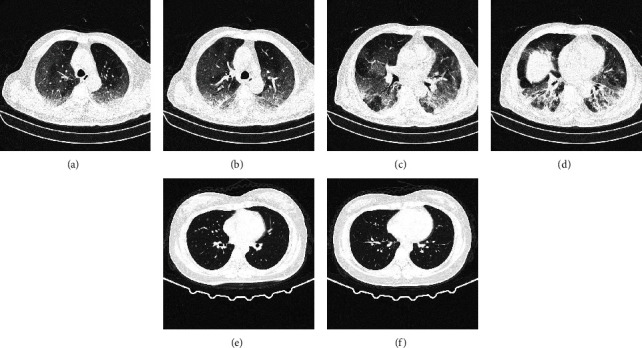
Examples of COVID-19 and non-COVID-19 CT images. (a–d) A 76-year-old male with confirmed COVID-19. (e–f) A 31-year-old female with non-COVID-19.
